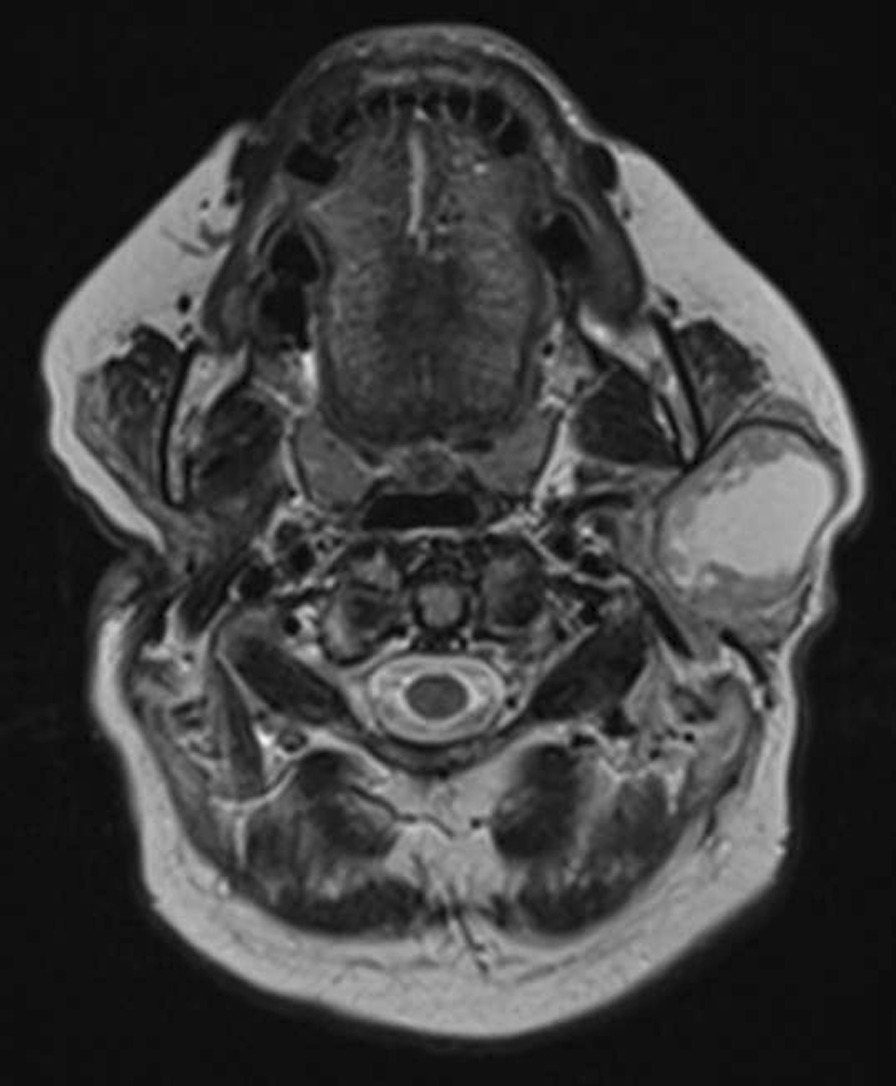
Axial T2-weighted MRI image post right total parotidectomy presenting a standard view of the post-operative site with one remaining lesion in the superficial lobe of the left parotid gland
